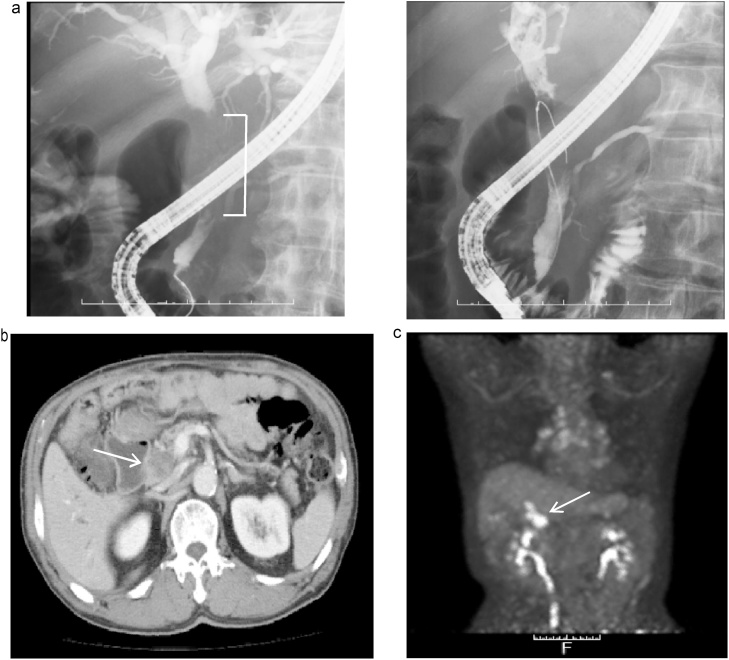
(a). Endoscopic retrograde cholangiopancreatography revealed a 5-cm-long disruption of contrast medium flow in the common bile duct, extending from the confluence of the cystic and common hepatic ducts to the distal bile duct (bracket), with significant dilation of the intrahepatic bile duct. Therefore, an endoscopic retrograde biliary drainage (ERBD) stent was inserted and placed. (b) A CT scan image revealed a contrast-enhanced protruding lesion that filled the lumen of the distal bile duct (arrow). This lesion did not extend beyond the wall of the bile duct, and neither infiltration into other organs nor clear lymphadenopathy was observed. (c) PET-CT scan revealed accumulation of FDG coinciding with the lesion in the bile duct (arrow), and no clear findings indicative of distant metastasis.
